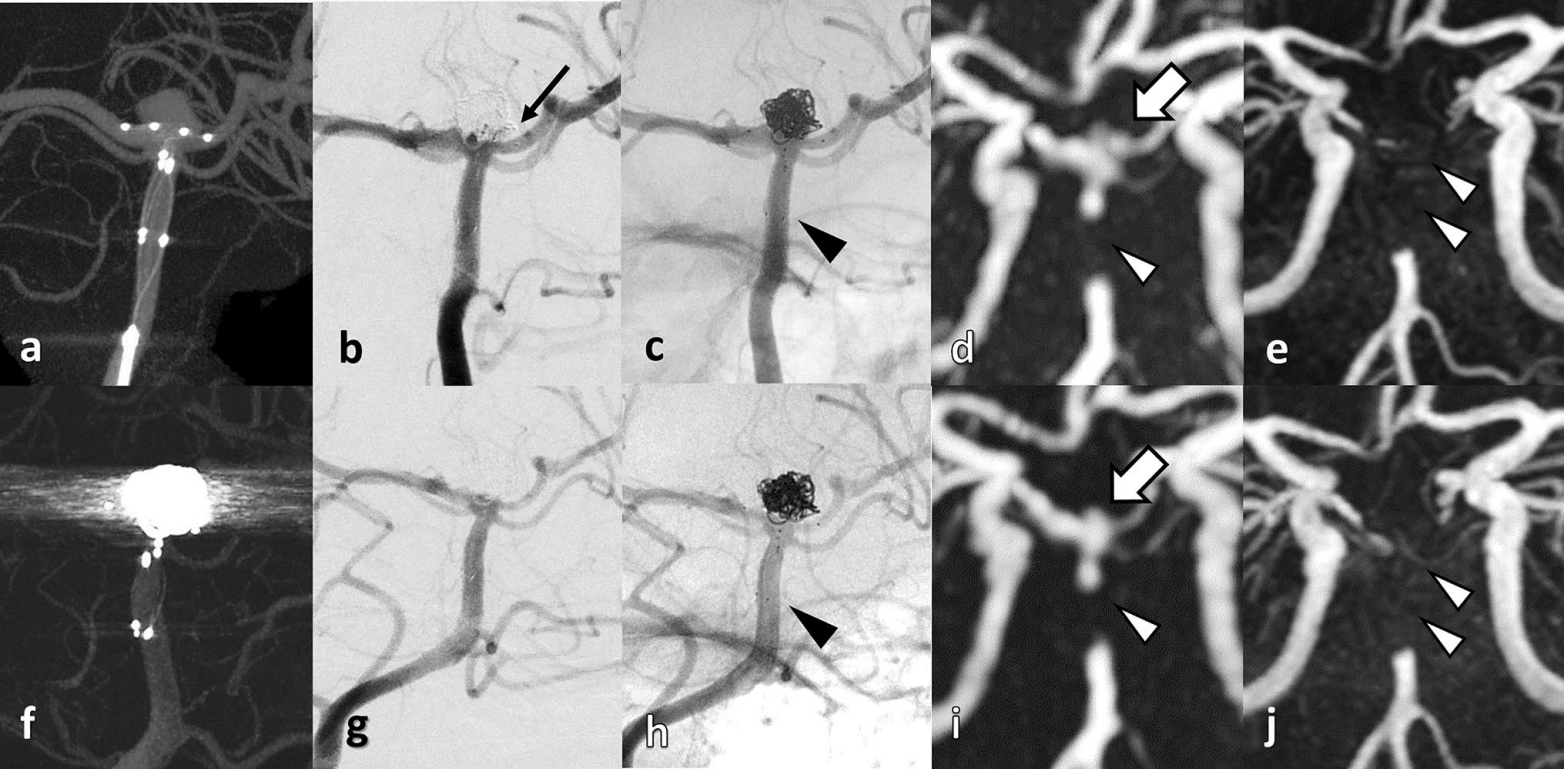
A 47-year-old female patient with an unruptured BA tip aneurysm (Case 3). a CBCT after PulseRider placement (T shape, 8 mm, 2.7–3.5 mm). b Postoperative DSA image after coil embolization with PulseRider. The black arrow indicates a small neck remnant. c Postoperative native DSA image. Black arrowhead indicates the proximal marker. d Silent MRA clearly shows a small neck remnant and parent artery in the bifurcation (white arrow). The segment of the proximal marker is poorly depicted (white arrowhead). e TOF-MRA failed to demonstrate the total area signal at the PulseRider placement location (double white arrowheads). Images at 6-month follow-up after PulseRider treatment. f CBCT of coiled aneurysm with PulseRider. g DSA image demonstrating complete occlusion of the aneurysm. h Native DSA image. Black arrowhead indicates the proximal marker. i Silent MRA image shows the occlusion of the residual neck similar to the DSA image (white arrow). The segment of the proximal marker is poorly depicted (white arrowhead). j TOF-MRA shows the same region with loss of signal as shown at the initial session (double white arrowheads). *BA* basilar artery, *CBCT* cone-beam computed tomography, *DSA* digital subtraction angiography, *MRA* magnetic resonance angiography, *TOF* time-of-flight
